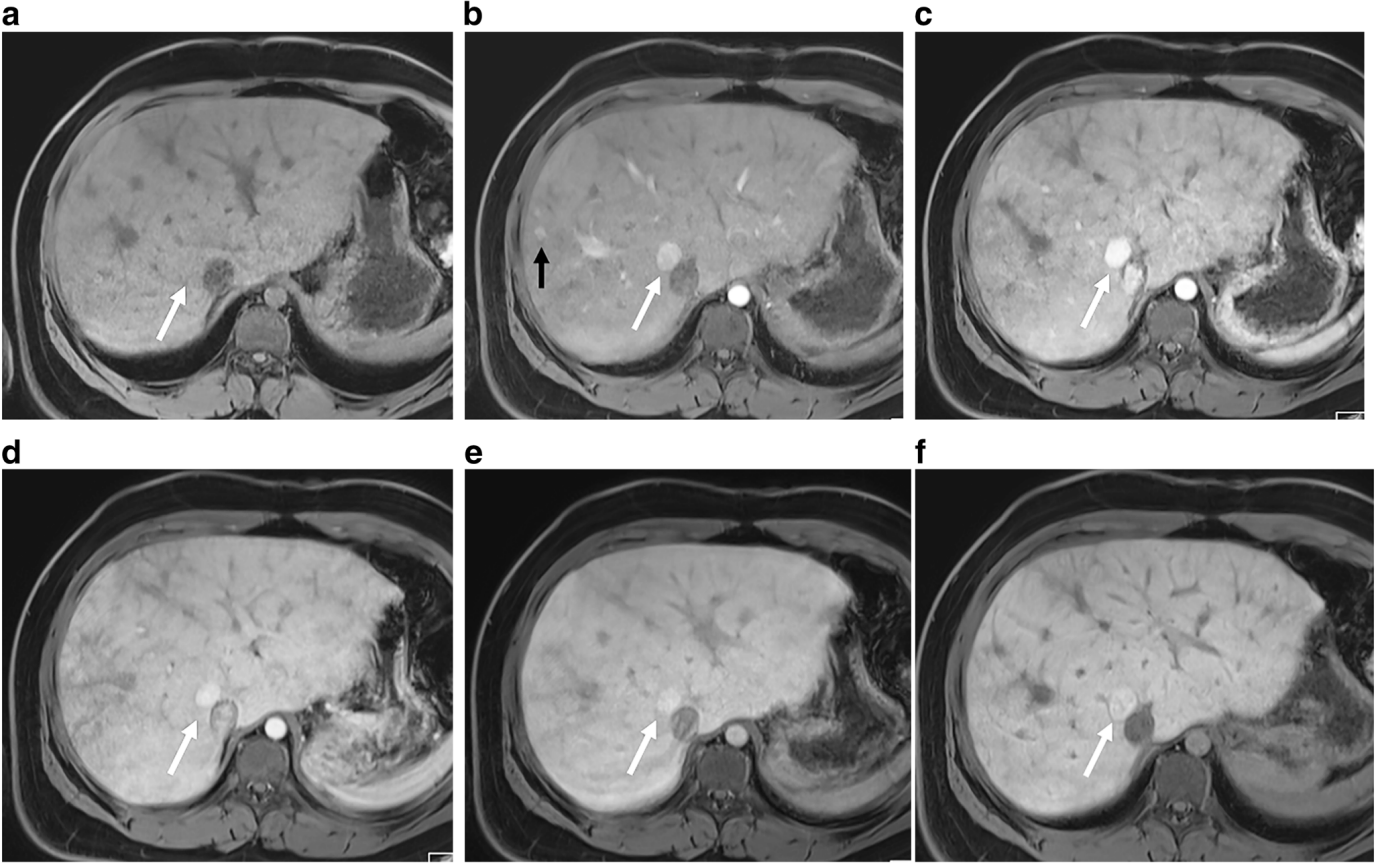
Tricuspid atresia and transposition of the great arteries in a 15-year-old girl with Fontan circulation and normal hepatic serological markers. As part of regular follow-up, MRI was performed including an axial gradient echo breath-hold sequence with fat suppression, sequences pre- (a) and post-injection of gadoxetic acid in arterial phase (b), portal venous phase (c), and after 2 min (d), 5 min (e) and 27 min (hepatobiliary phase) (f). Typical findings show an enlarged liver with an irregular congestive pattern. There is a nodular lesion anterolateral to the inferior caval vein (*white arrows*) with isointense signal to liver on T1-W pre-contrast and arterial enhancement with partial washout (e) and in hepatobiliary phase, a clear enhancement (f). The lesion was interpreted as a focal-nodular-hyperplasia-like lesion. Note additional small hypervascular nodules in the periphery (*black arrow* in b)
